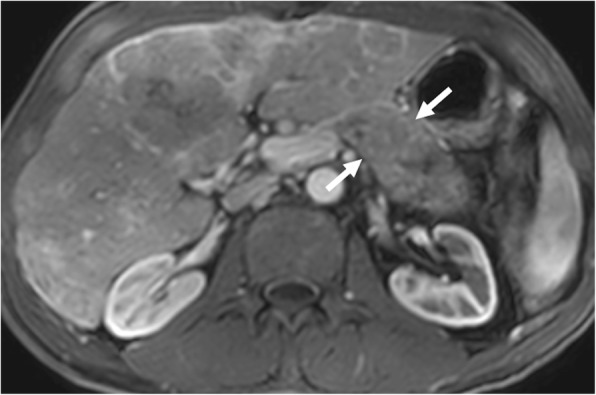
A 60-year-old male with known metastatic gastric NET. Axial plane portal phase postcontrast T1W MR image shows extensive tumor thrombus within the splenoportomesenteric venous junction extending into the splenic vein (arrows). Note that the endovenous tumor infiltrates beyond the confines of the splenic vein
